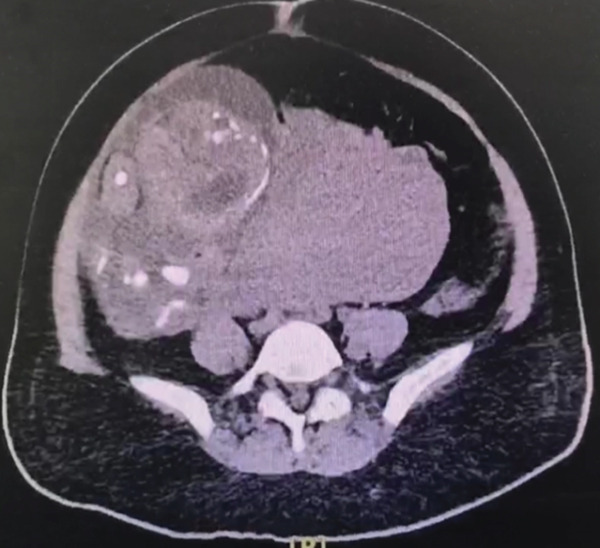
Abdominal CT demonstrating the intrauterine fetus, obtained during maternal evaluation for acute pancreatitis and metabolic derangements.
